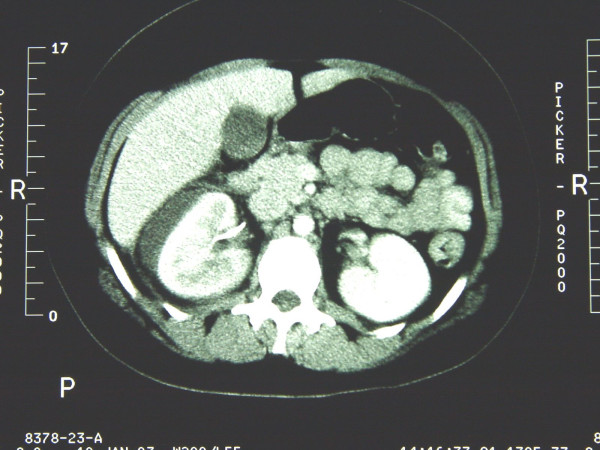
Abdominal CT scan with perirenal extravasation without evidence of abscess formation.
